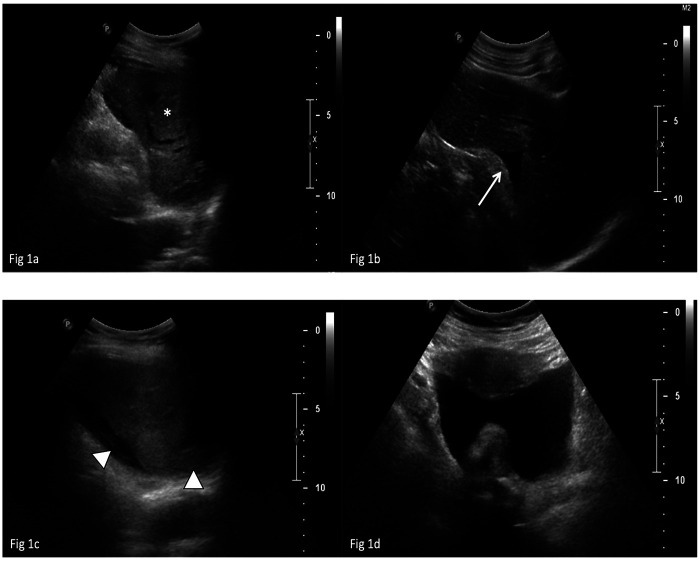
Abdominal ultrasound exam. In (a), a collection with heterogeneous, predominantly anechoic echotexture and hyperechoic strands is observed in the perisplenic region. A thin hypoechoic band surrounds a relatively hyperechoic central splenic area, raising suspicion of a focal lesion (*). In (b) and (c), the heterogeneous fluid collection (arrow) surrounds the spleen and contains hyperechoic strands (arrowheads in c). In (d), free heterogeneous fluid extends into the pelvic cavity.
